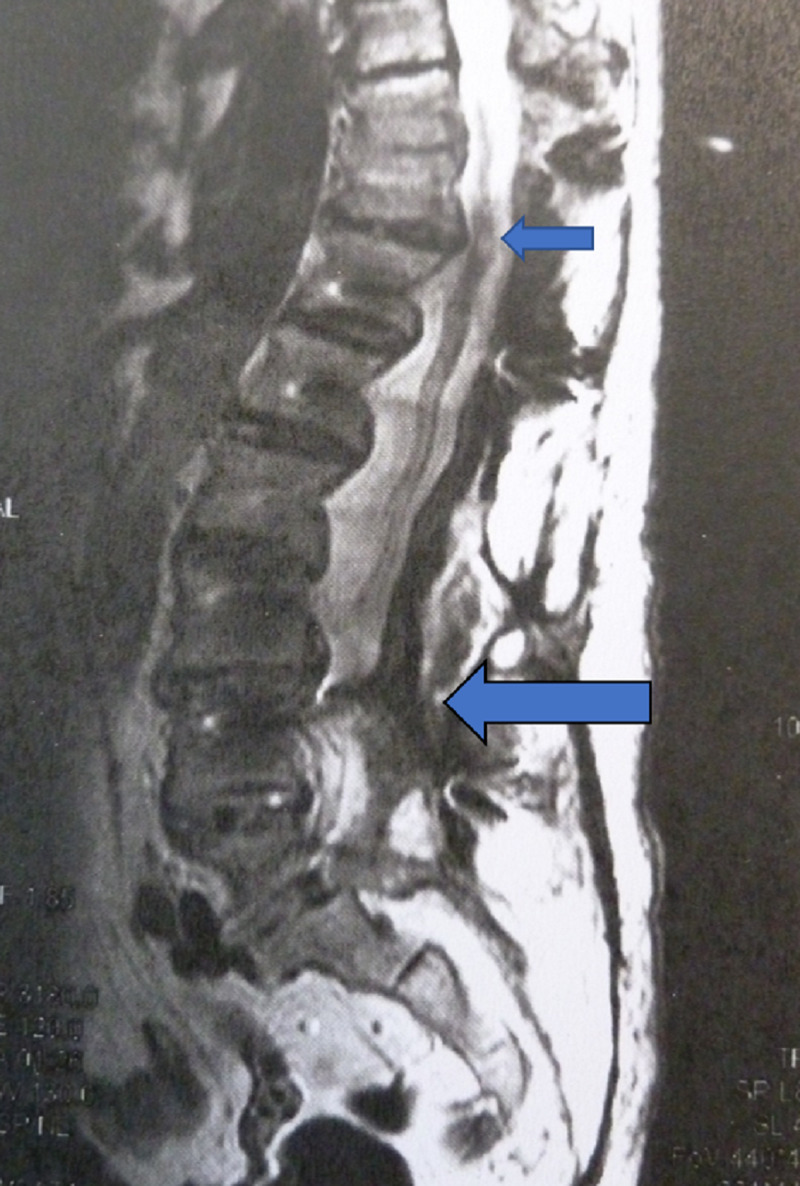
Lateral MRI showing myelopathy in the spinal cord at the level T11-T12 level (blue small arrow), Note significant dural adhesions at the level L3-L4 following MIS posterior decompression and fusion performed after primary XLIF (blue big arrow)
